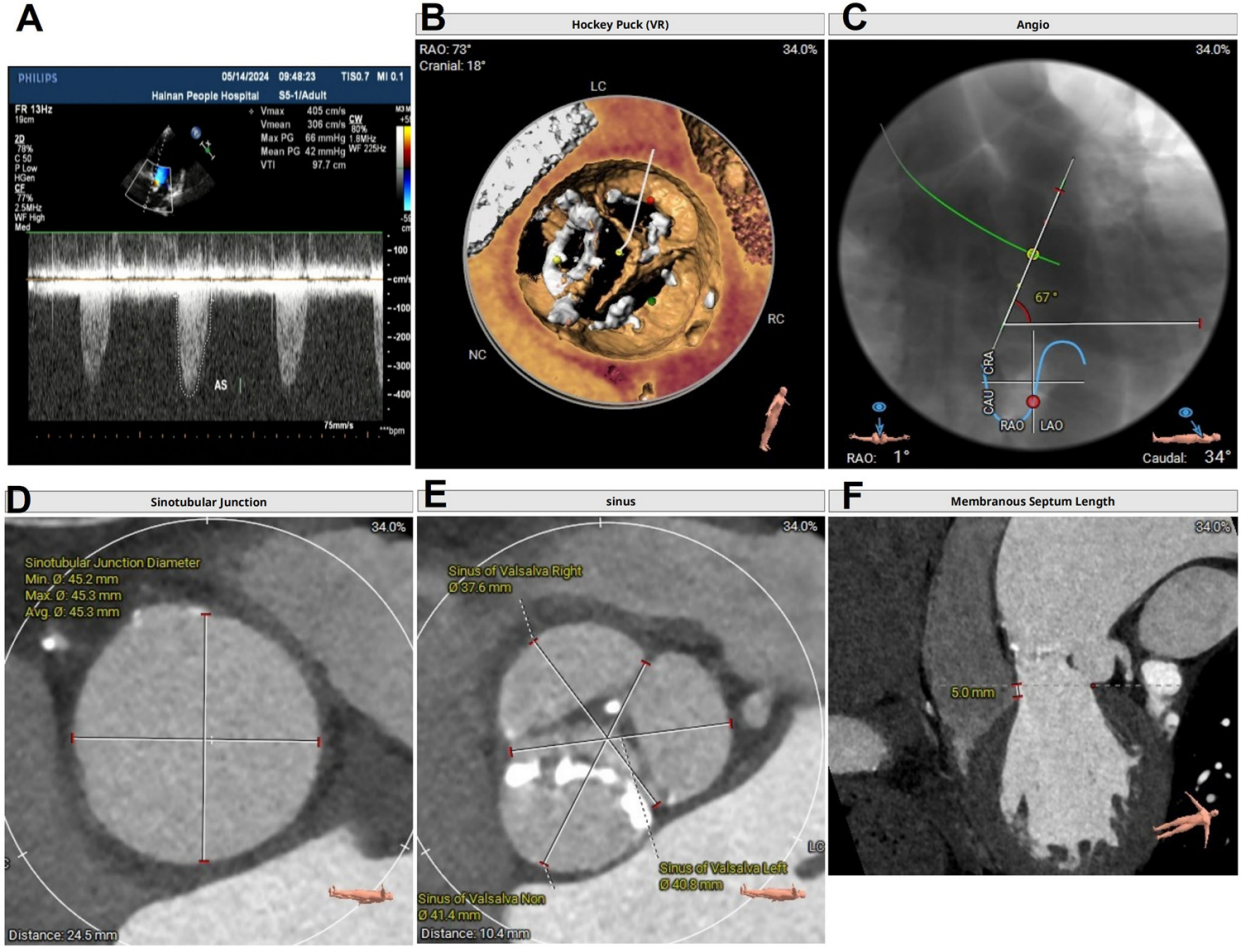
(A) Preoperative TTE in systole showing a mean gradient of 42 mmHg and a peak velocity of flow of 4.05 m/s, indicating severe AS; (B) preoperative aortic CTA showing a type I functional bicuspid valve; (C) preoperative aortic CTA indicating a horizontal heart position, with an angle of 67°; (D) a dilated ascending aorta; (E) a dilated sinus of Valsalva; and (F) the length of membranous septum was 5 mm. TTE, Transthoracic echocardiography; CTA, computed tomography angiography; AS, aortic stenosis.
